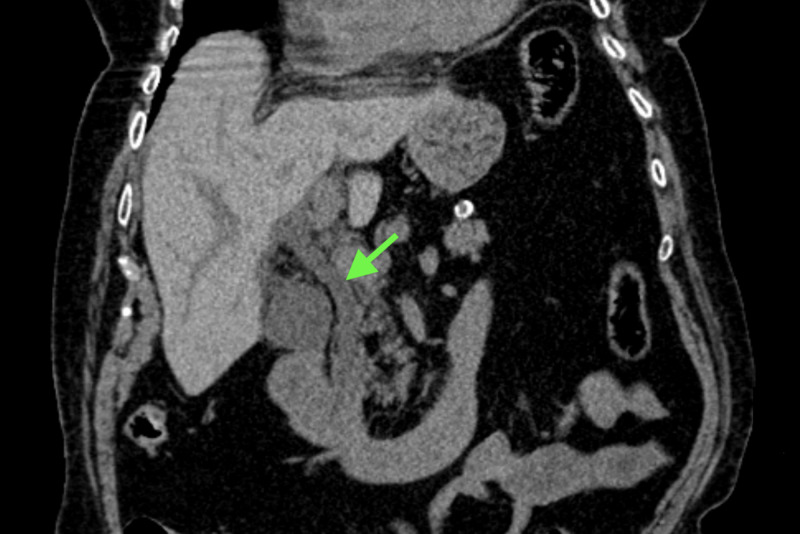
CT of the abdomen CT scan of the abdomen (coronal section) showing the dilated common bile duct (arrow) without clear peribiliary duct bile collection.
